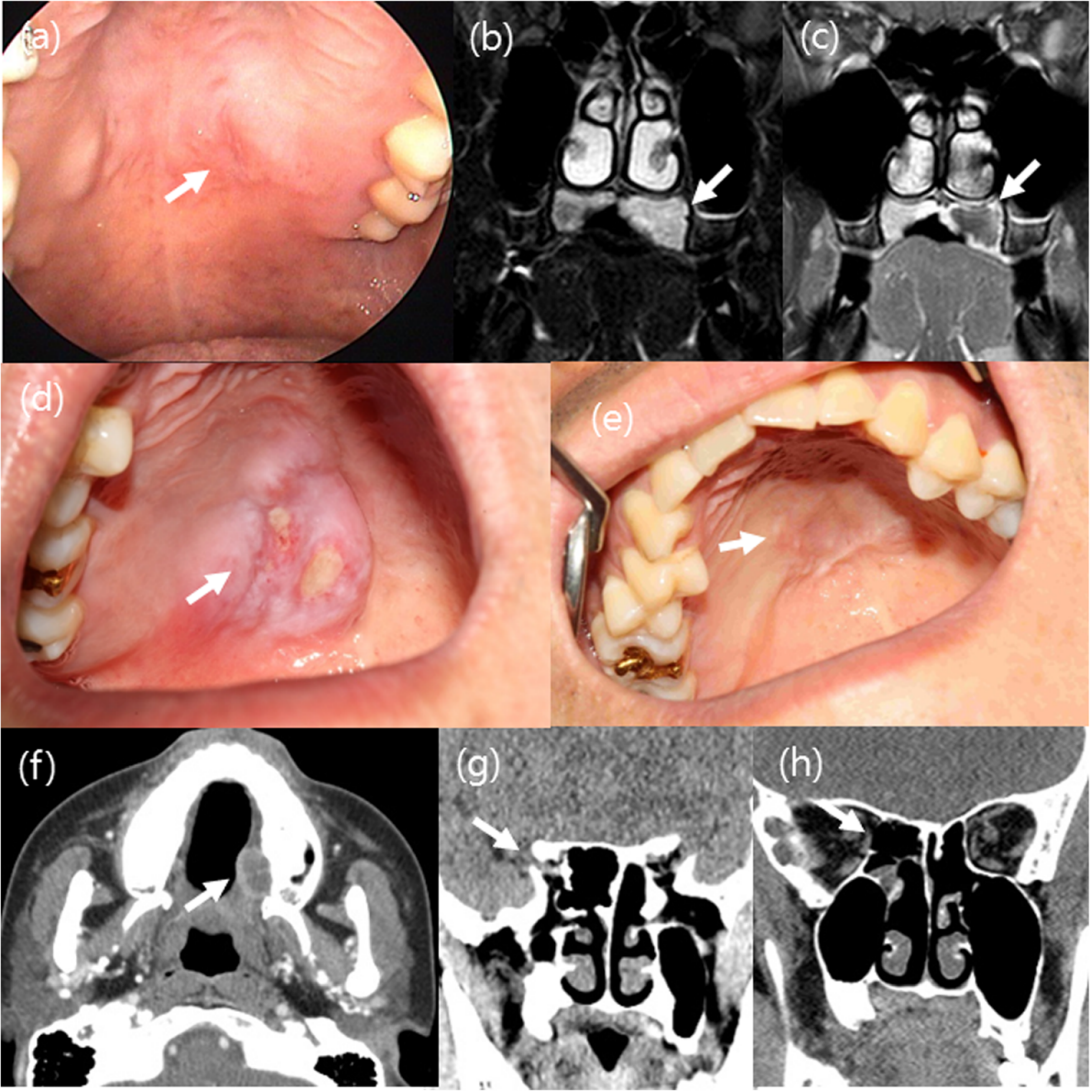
Clinicoradiologic findings of necrotizing sialometaplasia. In case 1, a bulging mass (a) in the left hard palate showing well defined high signal intentisity in T2 weighted coronal MRI (b) and peripheral enhancement and necrosis on post contrast T1-weighted coronal image (c). Case 3 showed an ulcerative mass (d) subsequently healed after 3 months (e). Case 2 also presented with a well demarcated peripheral enhancing mass in left hard palate by post contrast axial CT (f). Case 4 showed right sphenoid sinus wall defect without delineable mass in the nasal cavity or the palate (g). Two months after surgery, case 4 patient’s CT scan showed the same right sphenoid sinus wall defect (h)
